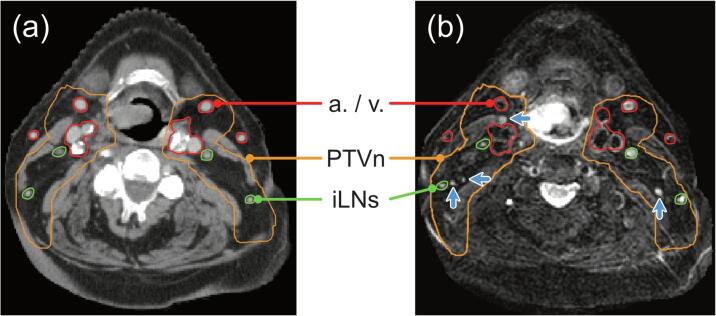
Comparison of lymph node visibility on CT and MRI. Corresponding transverse slices of a planning CT scan (a) and the water-only image of a T2-weighted MRI scan using mDixon fat separation (b). Blood vessels are indicated in red, conventional elective neck volumes (PTV_n_) in orange, and individual elective lymph nodes (i-LNs) in green. The blue arrows in (b) indicate four i-LNs that are not identified on CT, but that were found and contoured on the MRI scan in this slice. (For interpretation of the references to color in this figure legend, the reader is referred to the web version of this article.)
